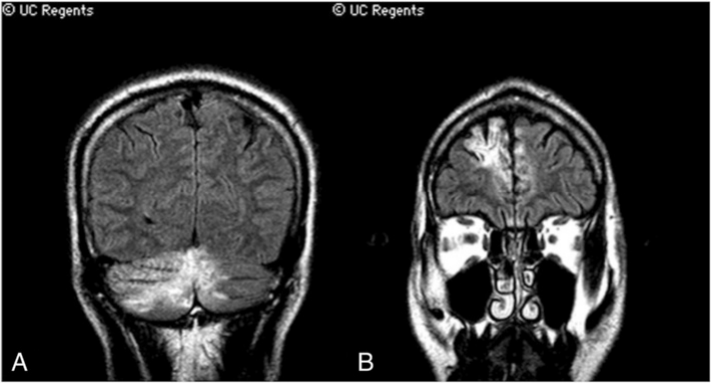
Magnetic resonance images from May 2003. Coronal fluid-attenuated inversion recovery (FLAIR) sequences through the posterior cerebellum (a) and the frontal lobe (b)
